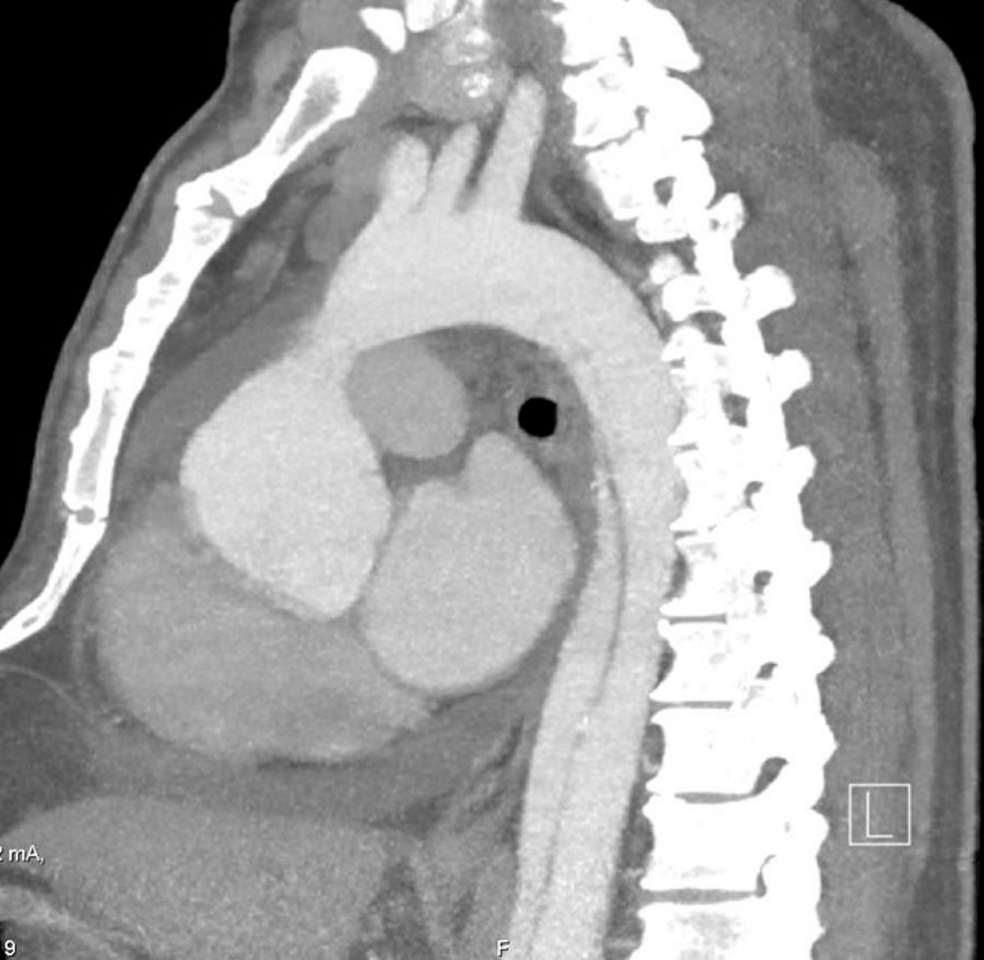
CT angiogram of the chest demonstrating the descending aorta double lumen
